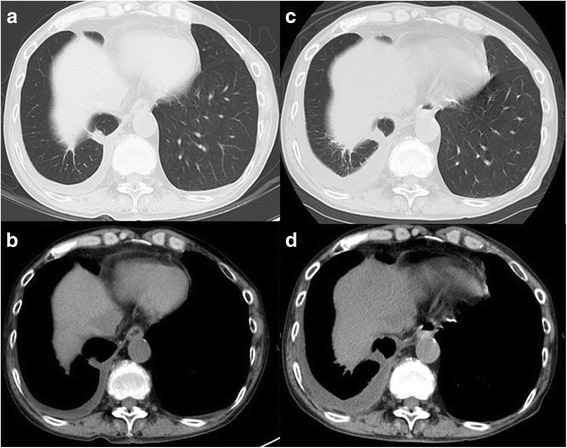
Images of CT after admission to the hospital. a and b on the day 8, (c) and (d) on the day 14. CT revealed an increased amount of pleural effusion
